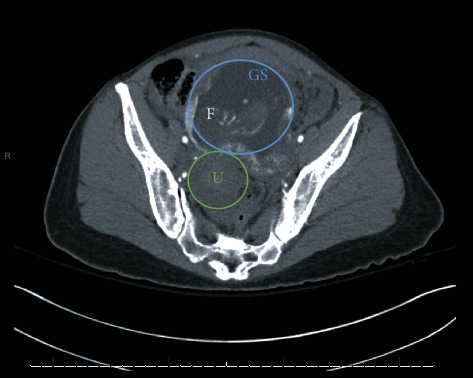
CTA showing fetal structures (F) within a gestational sac (GS) anterior to the uterus (U).
